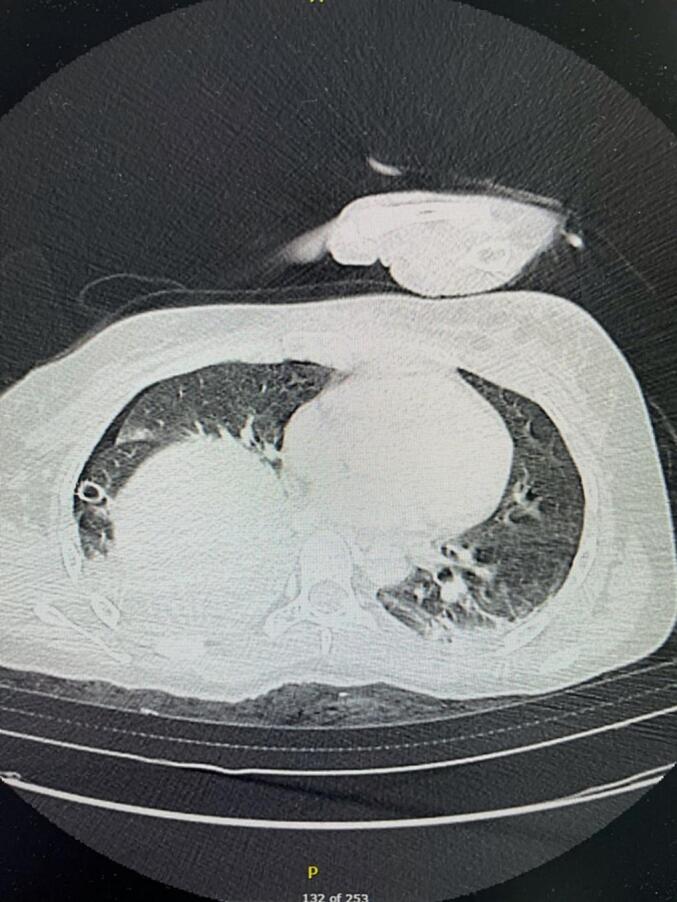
A CT scan in the transverse plane displays the right dome of the liver inside the chest cavity.
